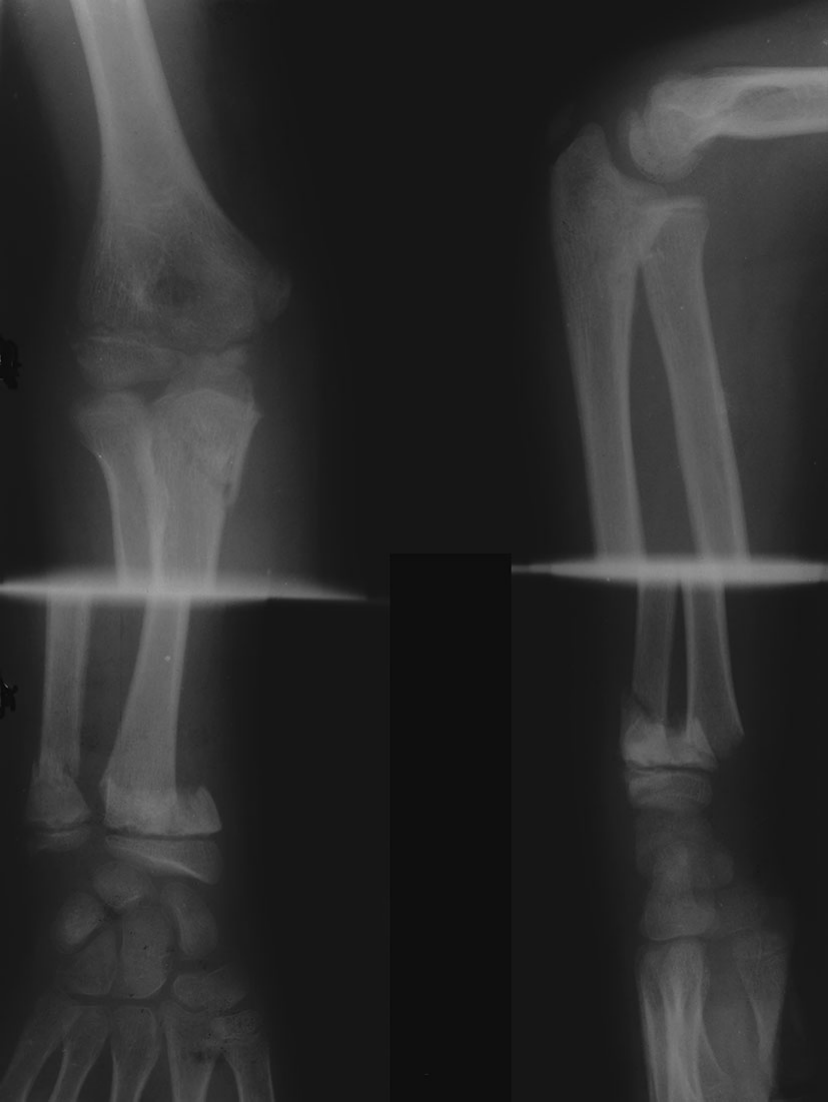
Radiographs of an 11-year-old boy with a type I segmental injury of the ulna. It presented as undisplaced fracture of the olecranon, and open displaced fractures of the distal part of the radius and ulna
